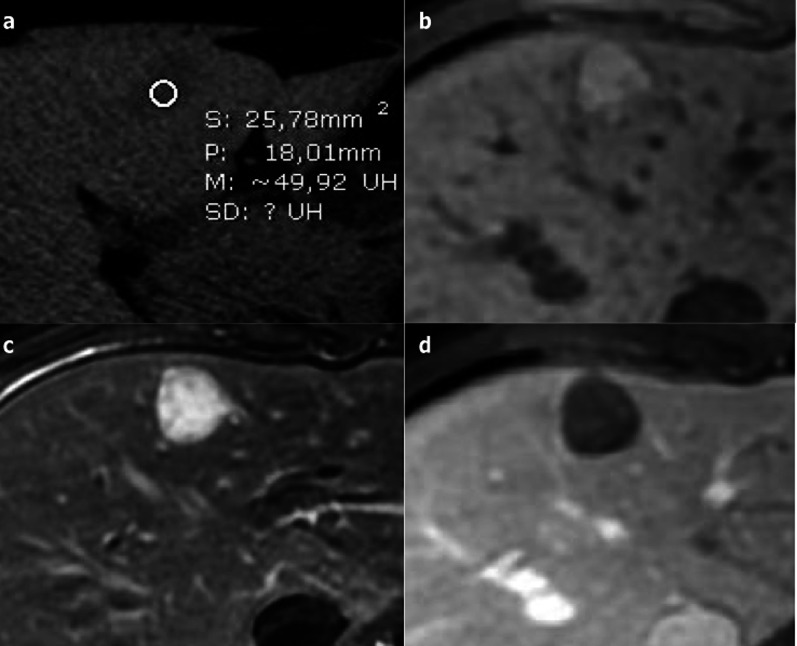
Ciliated hepatic foregut duplication cyst in a 15-year-old male. a Axial non-enhanced computed tomography shows a subcapsular nodule of the fourth segment with spontaneous attenuation value of around 50 Hounsfield Units. b On axial T1-weighted magnetic resonance imaging, it is spontaneously hyperintense. c It displays a high level of hyperintensity on axial T2-weighted magnetic resonance imaging. d Axial T1-weighted magnetic resonance imaging on portal venous phase shows neither contrast enhancement nor wall thickening
